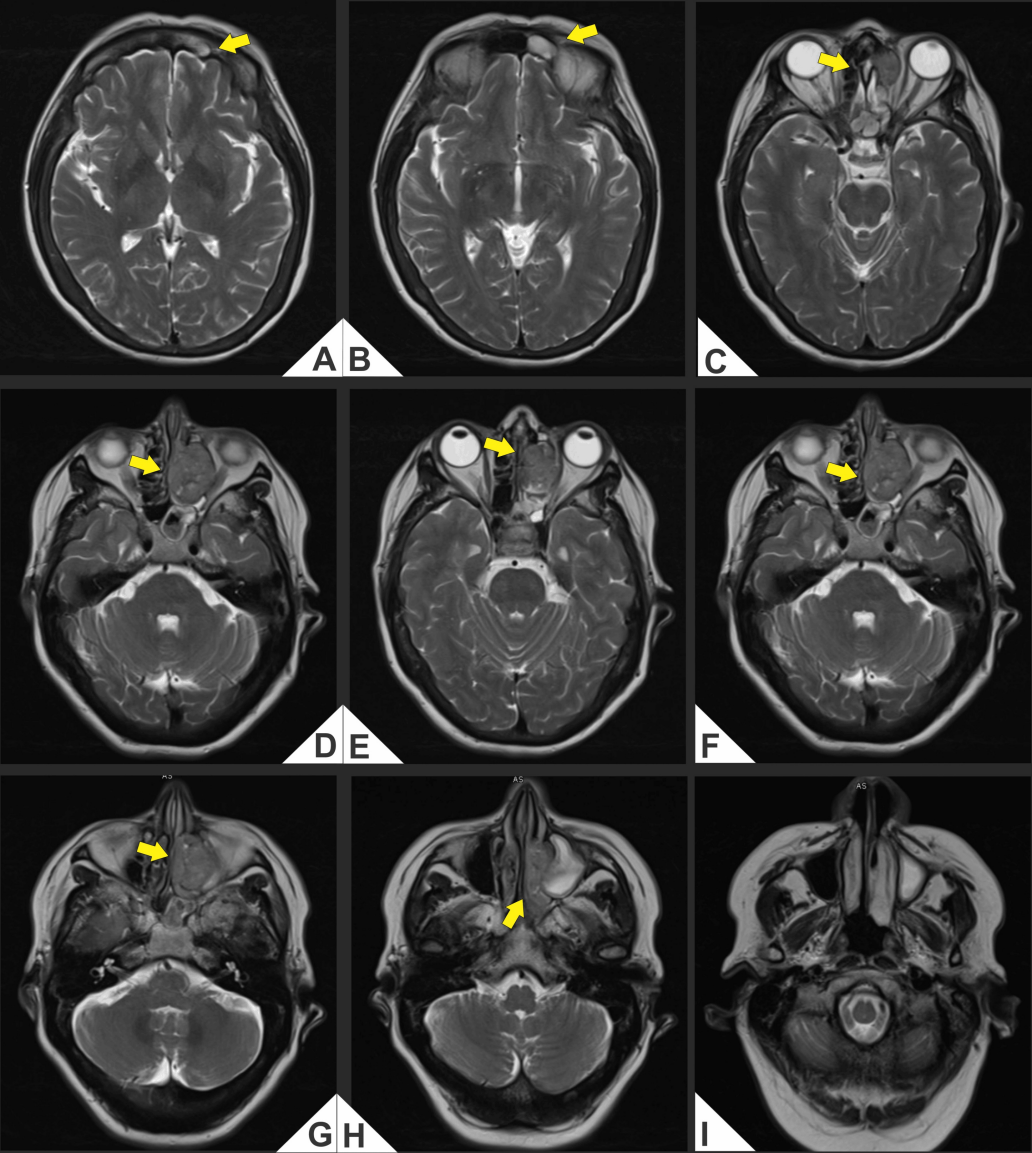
(A-I) Axial T2-weighted magnetic resonance imaging (MRI; non-fat-suppressed). Lobulated T2-hyperintense mass centered in the left nasoethmoid region, measuring 3.24 × 2.47 × 4.22 cm (craniocaudal × anteroposterior × transverse), with medial extraconal orbital extension that molds the medial rectus and effaces the adjacent extraconal fat, without definite intraconal involvement. (A, B) Effacement of the subfrontal fat plane with abutment of the cribriform plate/anterior skull base; (C-H) Persistent heterogeneous T2 signal with internal hypointense foci and inferior extension toward the ethmoid-meatal recess and the medial wall of the left maxillary sinus. Findings are concordant with Kadish C topography and with lamina papyracea erosion documented on CT (Figure 1 and Figure 2).
